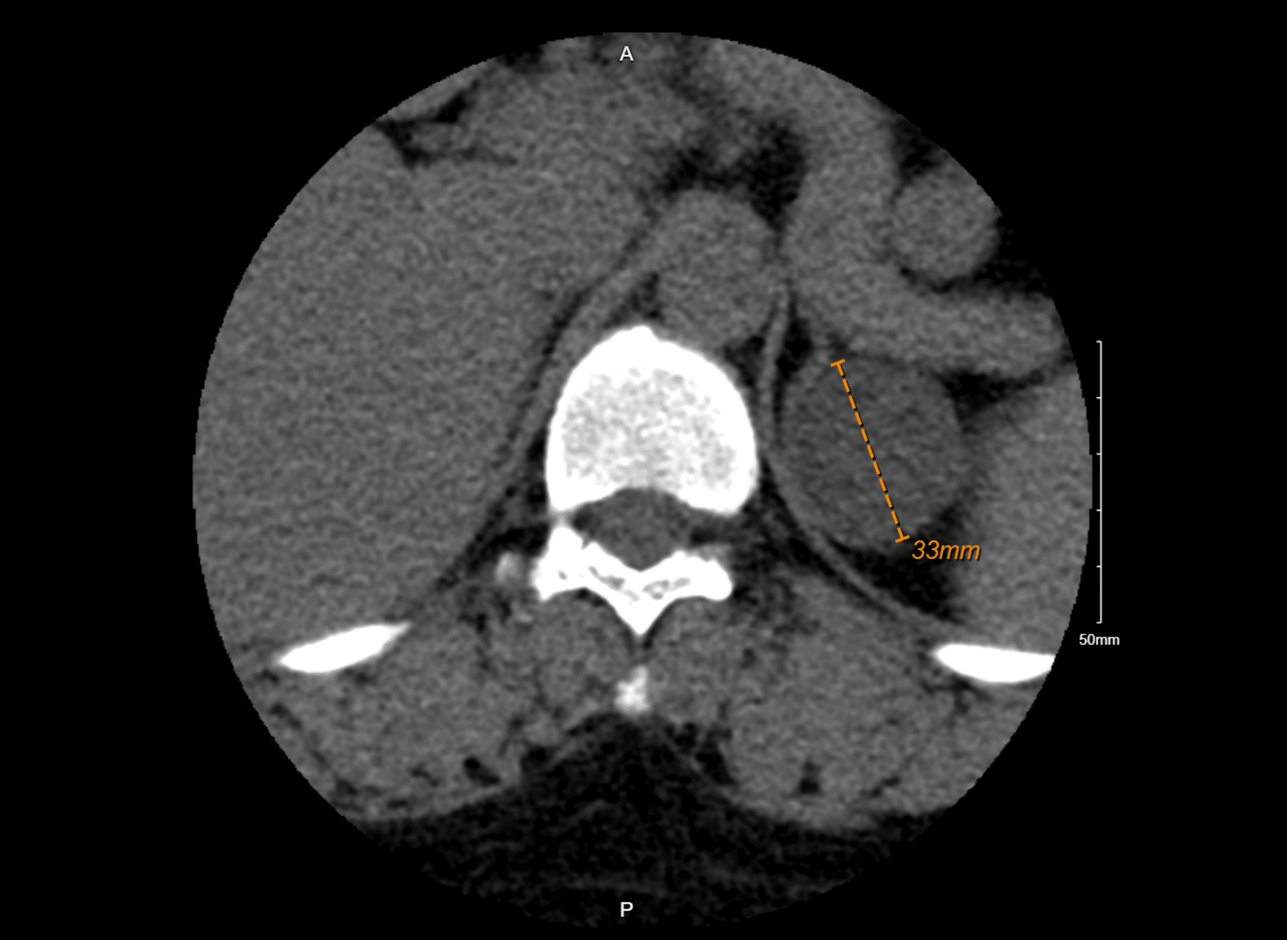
CT lumbar spine showing a 3.3 cm low-density cystic mass arising from the left adrenal gland versus the superior pole of the left kidney
